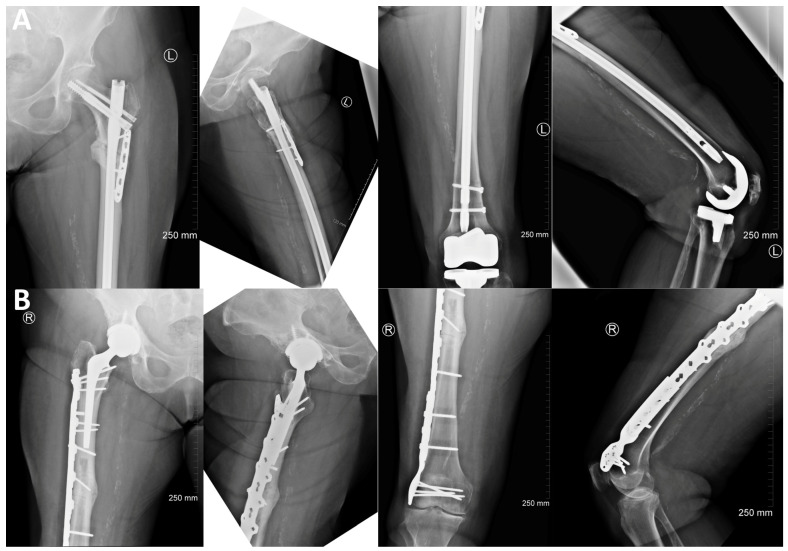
Most recent follow-up radiographs demonstrating healing of both femur fractures with no evidence of hardware failure: (A)—approximately 10 months following left femur nonunion surgery (top) and (B)—2 years after right atypical periprosthetic femoral shaft fixation (bottom).
